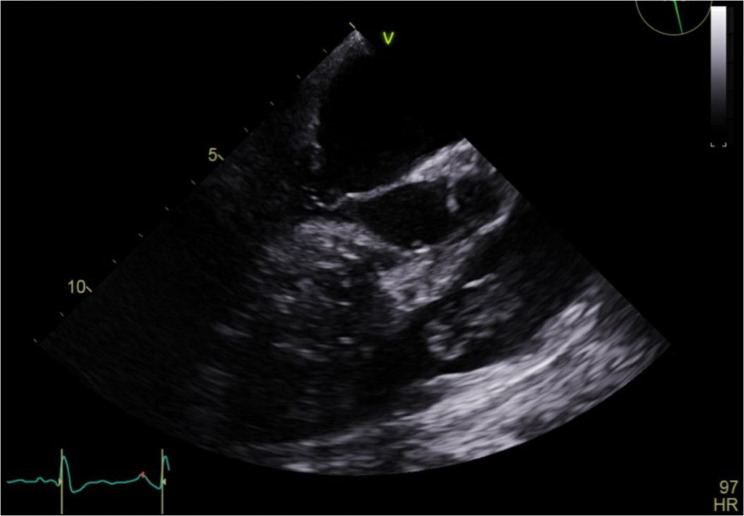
Transesophageal echocardiogram. Mid-esophageal short-axis. 2.0 × 0.8 cm mass suggestive of a vegetation adhered to the pulmonary valve. Mildly dilated right ventricle
